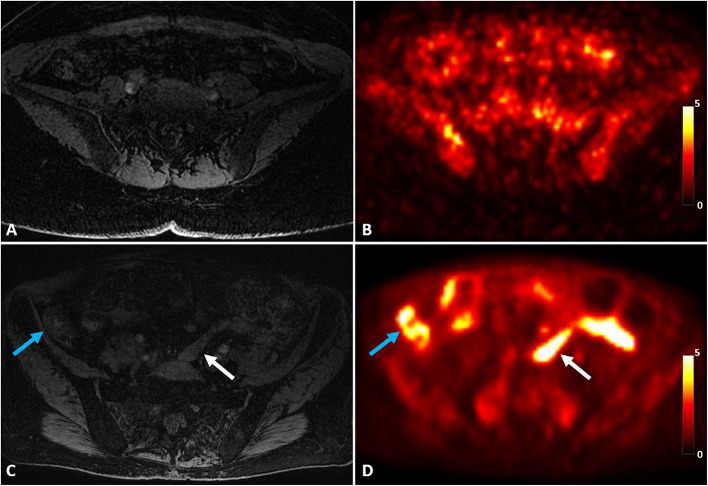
A representative control case of MRI (A) and S1R PET (B) of a healthy control in comparison with abnormal pelvic bowel uptake of Patient 2 on MRI (C) and S1R PET (D). Increased S1R tracer uptake was identified throughout the bowel, including both within the bowel (white arrow) and around the bowel (blue arrow). The PET images were plotted in the unit of SUV.
